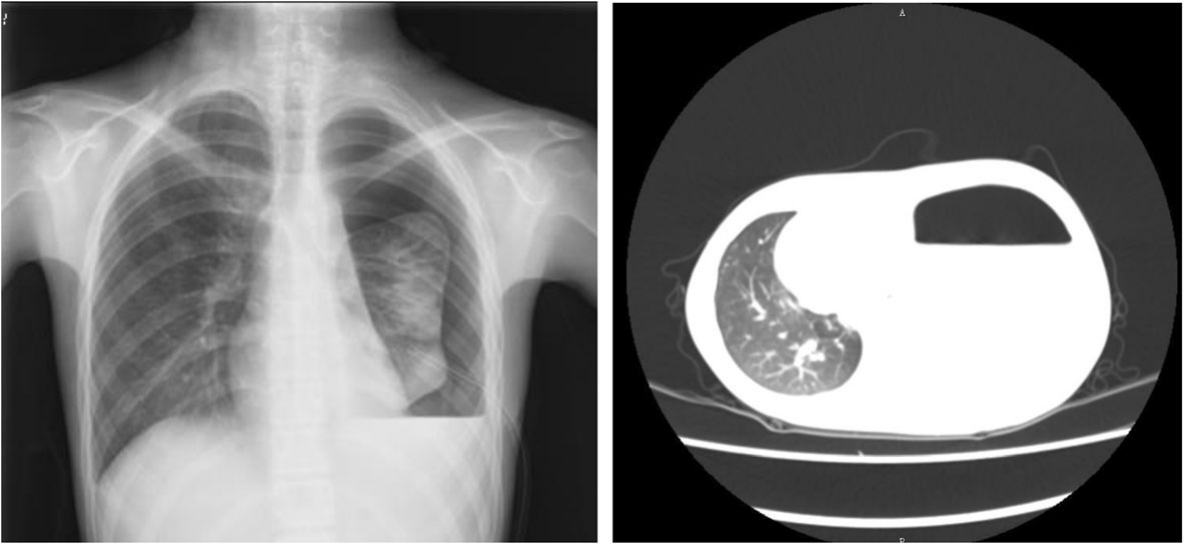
An air-fluid level and atelectasis can be seen on the chest x-ray (left) and computed tomography (right) images, which showing massive left hydropneumothorax
